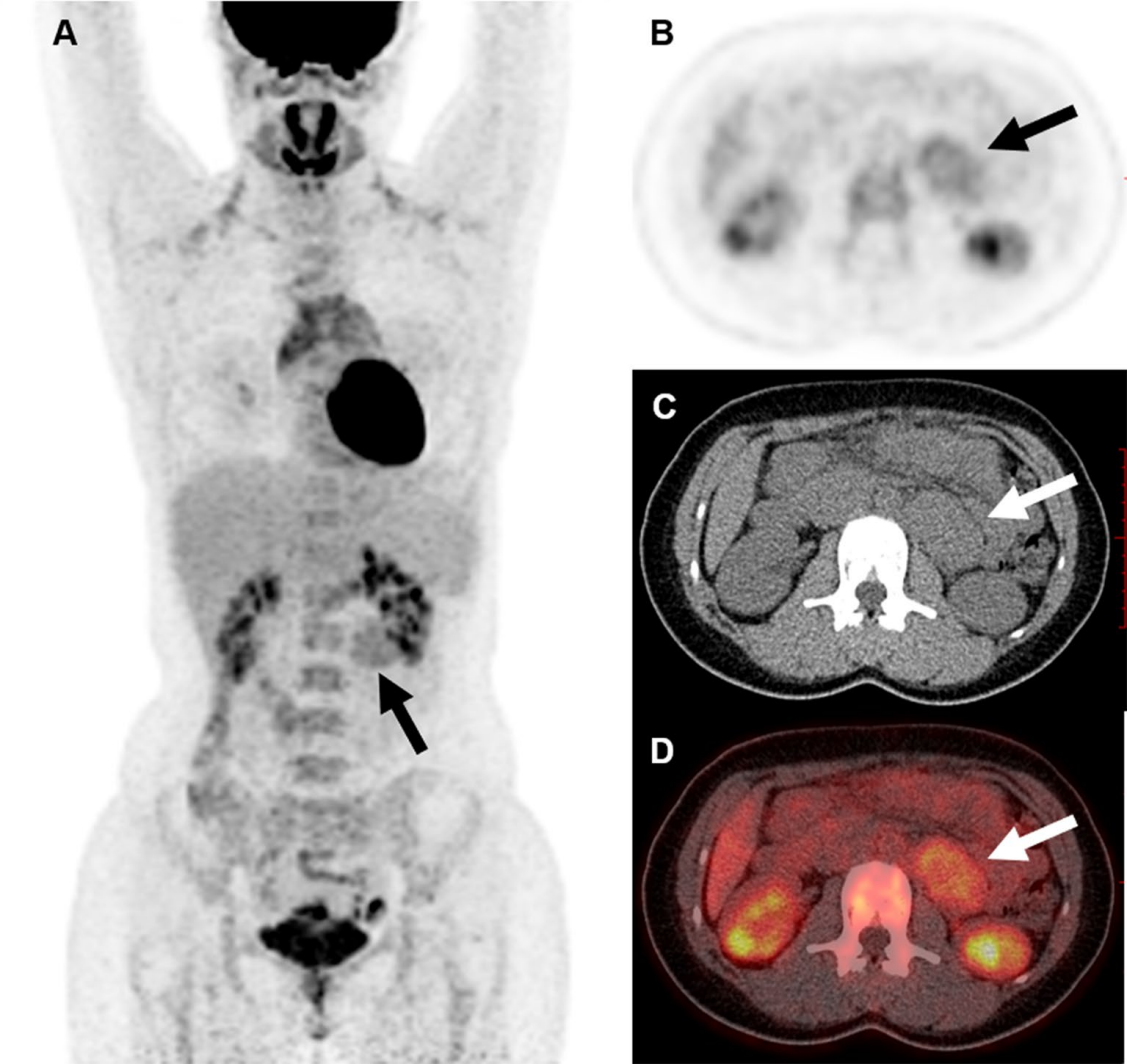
Representative case of a 25-year-old woman who underwent ^18^F-FDG PET/CT to evaluate a retroperitoneal solid mass (patient 27). The mass demonstrated moderately increased FDG uptake with an SUVmax of 3.2 and a size of 5.0 cm (arrows). Hypermetabolism was also seen in bilateral cervical and anterior mediastinal brown fat. The pathology confirmed the diagnosis of a Castleman’s disease, the hyaline vascular variant.
